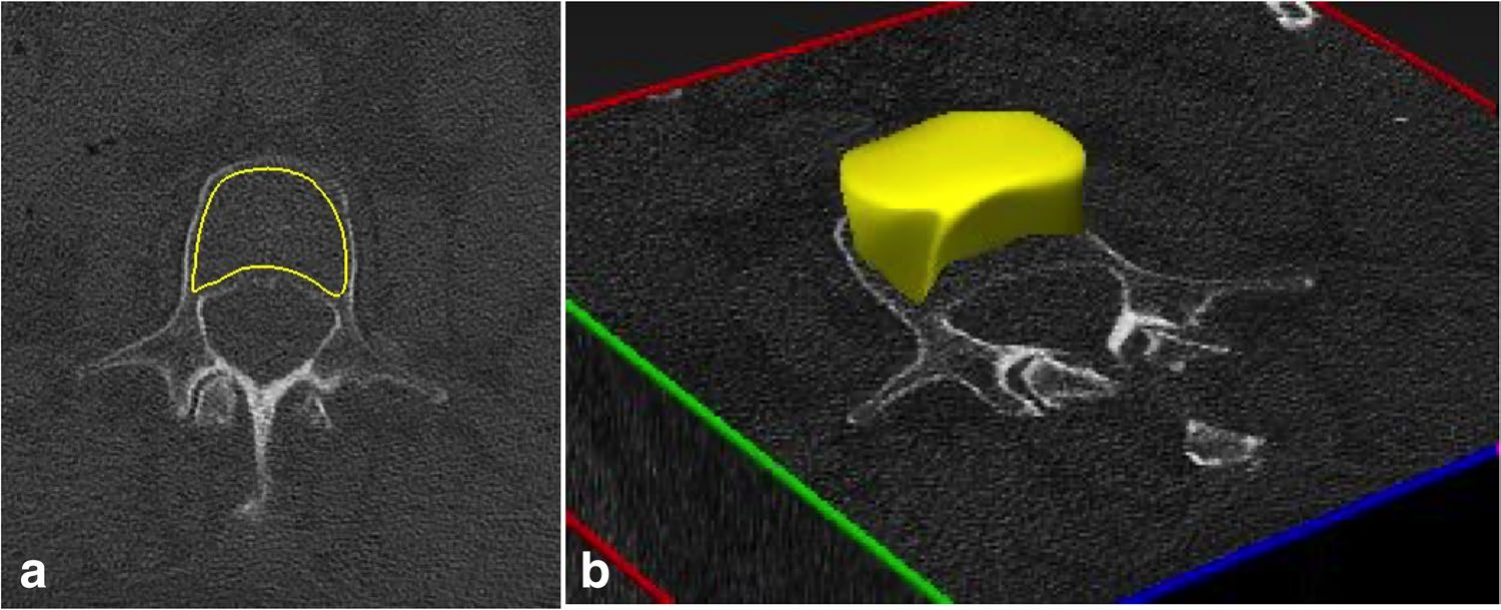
Manual definition of the trabecular volume of interest (VOI) by one reader (C. Booz, radiology resident with 4 years of experience in musculoskeletal imaging) using specific DECT postprocessing software (LiverLab; Fraunhofer IGD) (a). This was repeated throughout the entire stack of 2D slices for each vertebra. The resulting three-dimensional VOI (b) served as input for volumetric DECT BMD assessment in combination with the two DECT series (90 and Sn150 kVp). VOI, volume of interest; DECT, dual-energy CT; BMD, bone mineral density
